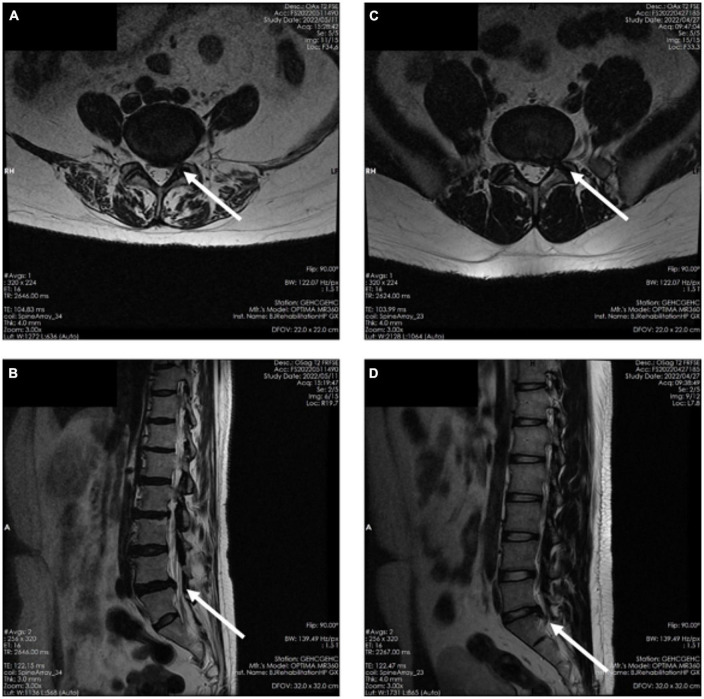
(A) Magnetic resonance imaging axial planes in patients with L4/L5 nerve root compression. (B) MRI sagittal planes in patients with L4/L5 nerve root compression. (C) MRI axial planes in patients with L5/S1 nerve root compression. (D) MRI sagittal planes in patients with L5/S1 nerve root compression planes.
